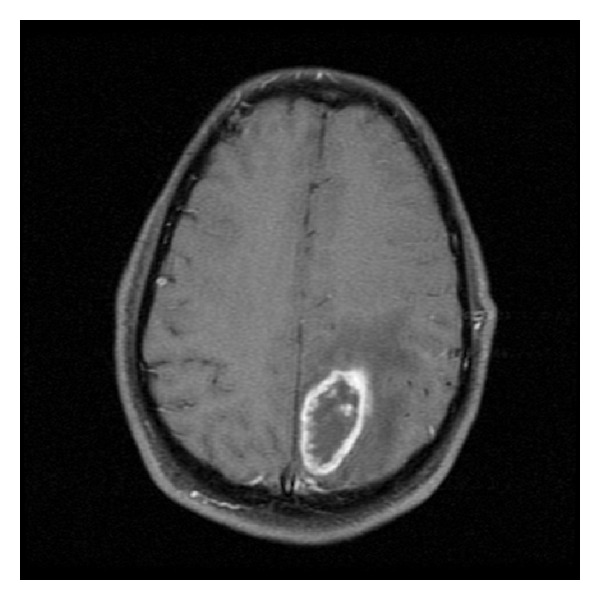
MRI scan obtained after this patient initially presented to the emergency department demonstrating 4.5 cm, ring-enhancing, left parietal mass with surrounding edema consistent with GBM.
